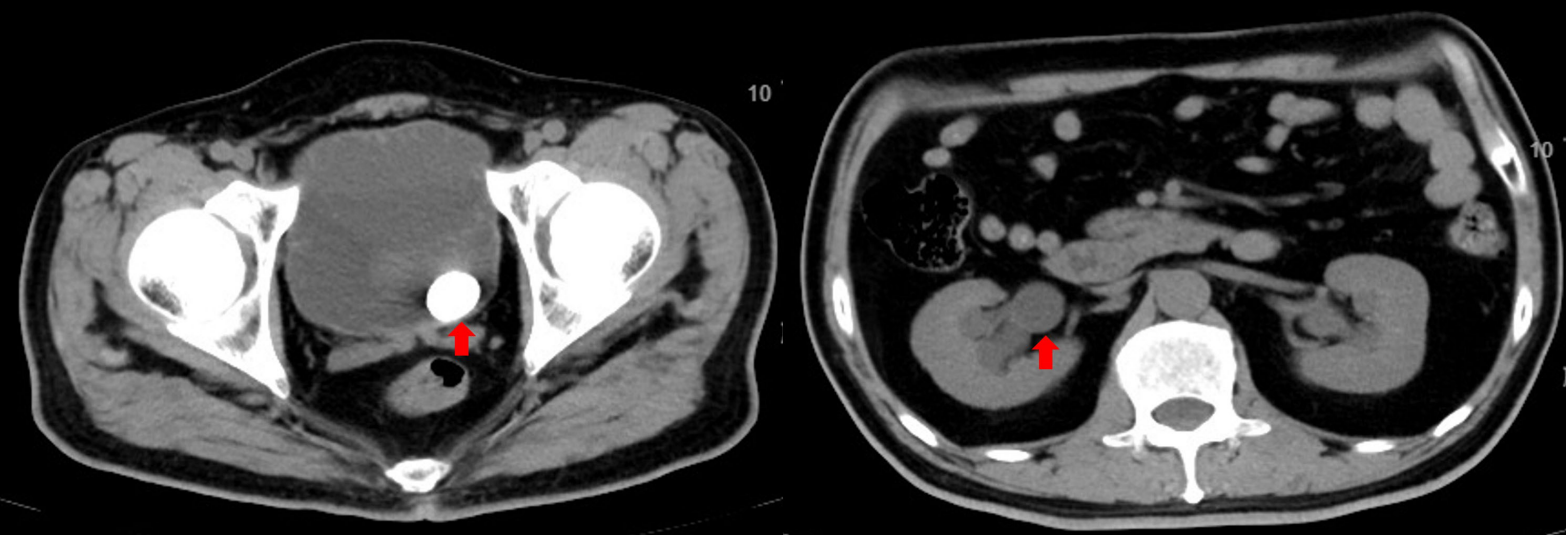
Abdominal computed tomography. Both kidneys were normal in position and size with regular morphology. Bilateral renal parenchyma demonstrated round hypodense lesions, the largest measuring approximately 9 mm in diameter. Bilateral pelvicalyceal systems and ureters were dilated with fluid accumulation. Bilateral perirenal fat planes were clear. The bladder was overdistended, containing a nodular hyperdense lesion measuring approximately 23 mm × 18 mm; a small diverticulum was noted on the right lateral wall. The prostate was enlarged with a diameter of approximately 54 mm, demonstrating punctate hyperdense foci and protruding into the bladder. Bilateral seminal vesicles were symmetrical with homogeneous density.
